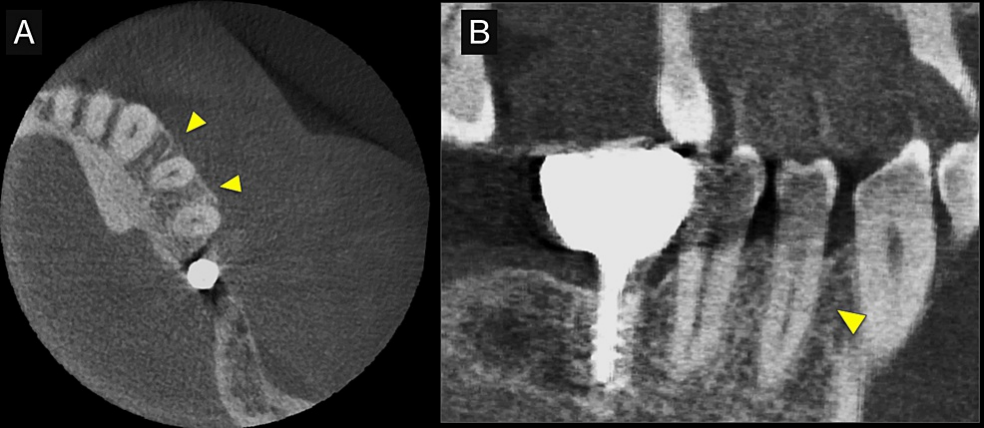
Postoperative cone-beam computed tomography (CBCT) images. (A) CBCT axial view, and (B) CBCT oblique sagittal view. CBCT images showed the bone-like structures formed in the intrabony defect area (arrowheads).
